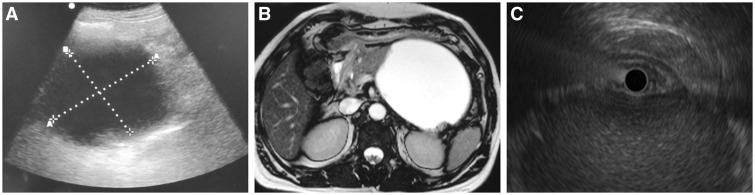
Imaging in patient with WOPN having <10 % solid necrotic debris. a) Abdominal ultrasound; b) Magnetic resonance imaging; c) Endoscopic ultrasound.
